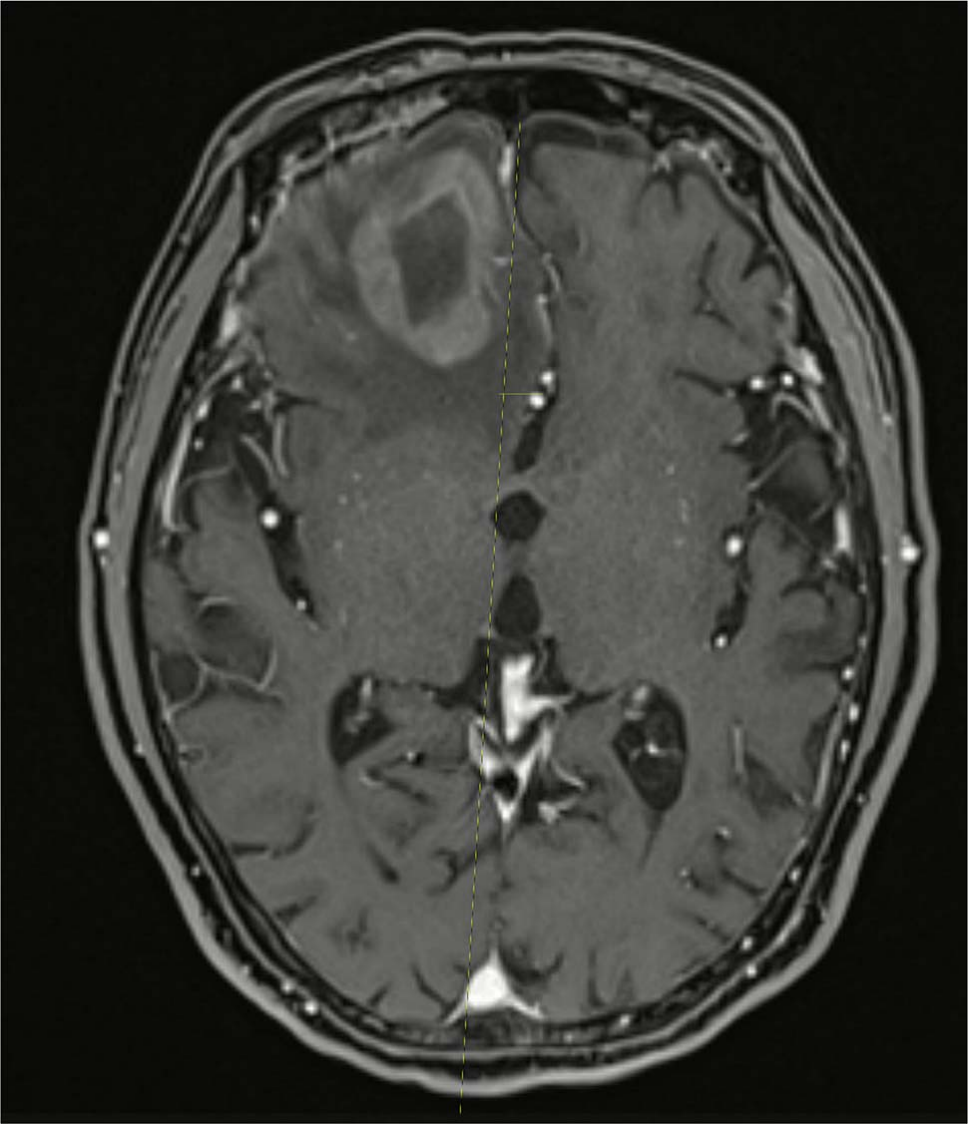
MRI brain showing the right frontal lobe mass causing midline shift.
